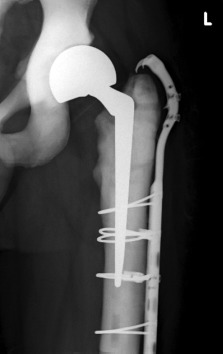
Radiograph 2 years post-operative.
